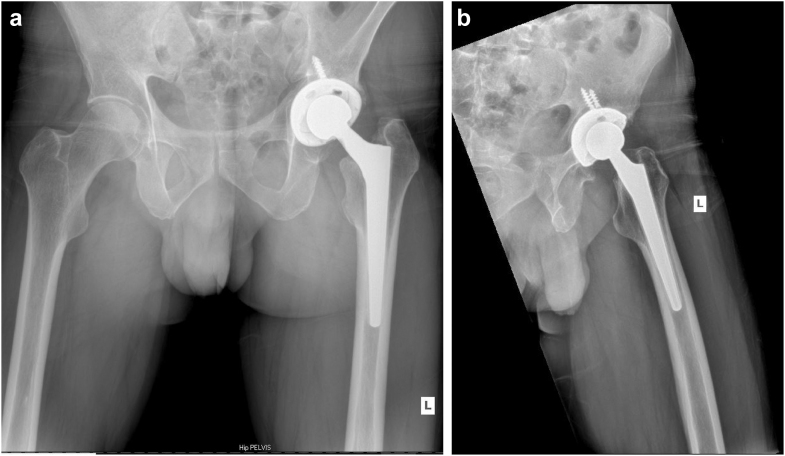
(a) AP pelvis X-ray of the left hip showing good alignment of the implants. (b) Lateral X-ray of the left hip showing good alignment of the implants.
